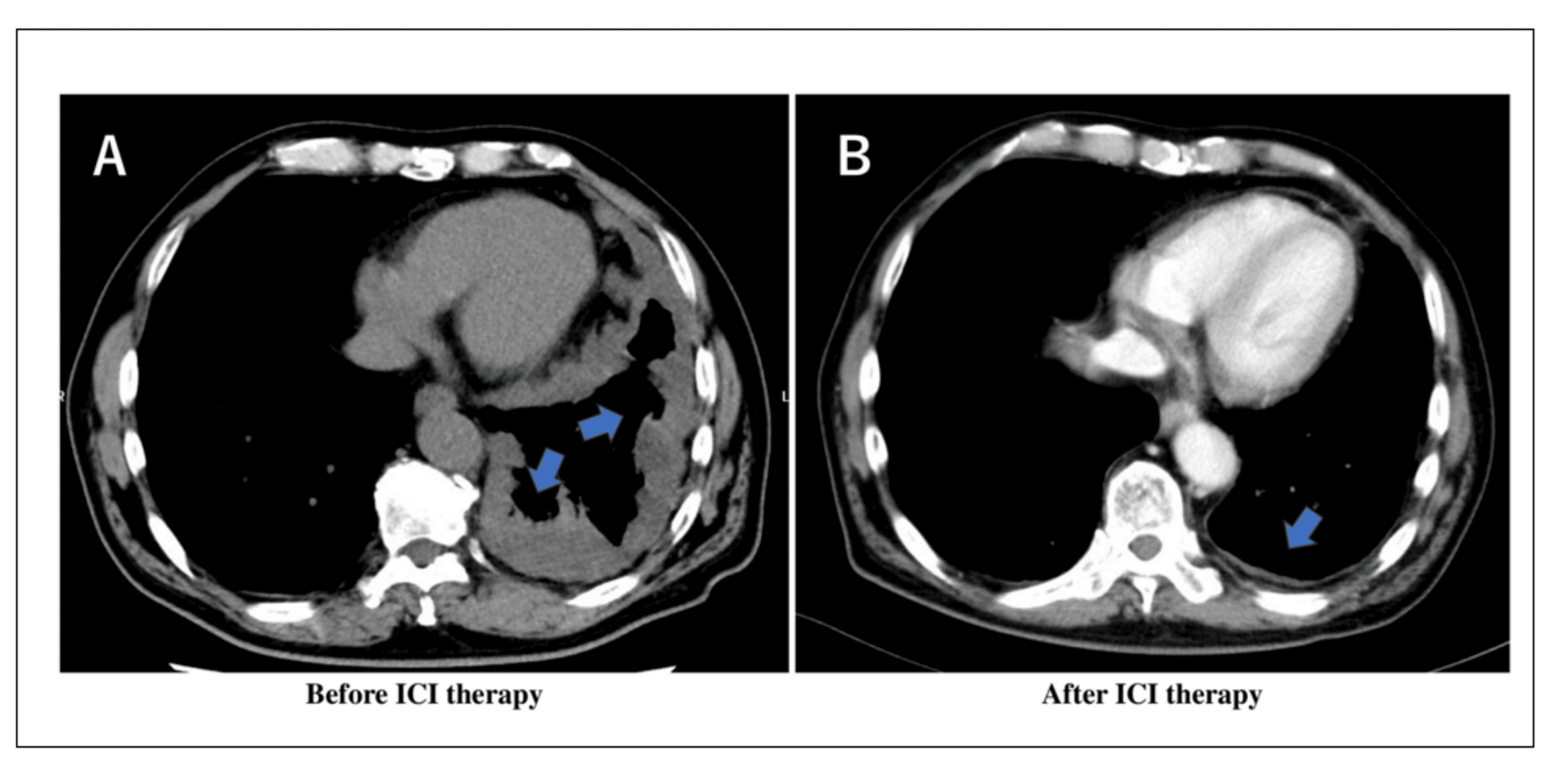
Chest CT image before and after ipilimumab and nivolumab combination therapy (A) Chest CT image before the initiation of ipilimumab and nivolumab combination therapy. (B) Chest CT image after three courses of ipilimumab and nivolumab combination therapy. ICI: Immune checkpoint inhibitor.
